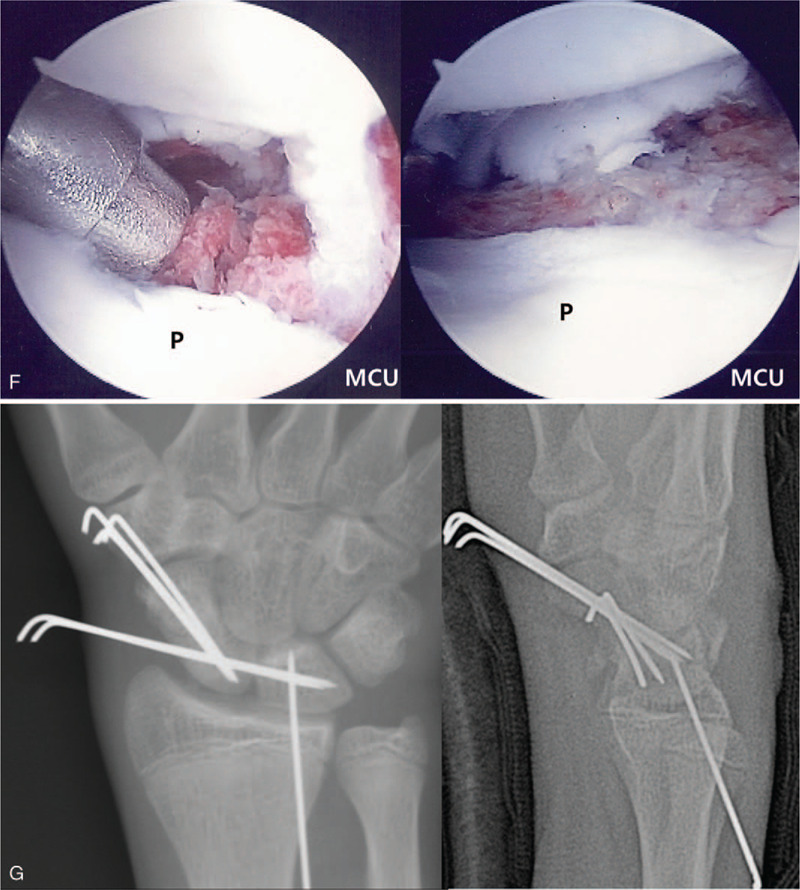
A 15-year-old boy patient with nonunion of the right scaphoid fracture. (A) Preoperative right wrist plain PA with ulnar deviation view showing nonunion at the waist of the scaphoid. (B) Preoperative right wrist sagittal CT scan shows nonunion at the waist of the scaphoid, about 88 degrees lateral intra-scaphoid angle and a humpback deformity. (C) Mid-carpal arthroscopy image of scaphoid nonunion site (P: proximal fragment, D: distal fragment). (D) Mid-carpal arthroscopy image of nonunion site after debridement showing punctate bleeding from proximal and distal fragments. (E) Mid-carpal arthroscopy image of SL joint showing volar side instability (Sc: scaphoid, Lu: lunate). (F) A mid-carpal arthroscopy image of percutaneous autogenous iliac cancellous bone grafting at the nonunion site using cannula and trocar (left) and complete the bone graft (right). (G) Immediate postoperative plain right wrist PA with ulnar deviation and lateral radiographs show internal fixation with K-wires and grafted bone at the nonunion site. (H) Postoperative 9 weeks K-wires removal axial and sagittal CT scans show complete bony union and correction of humpback deformity. (I) At 54 months’ follow-up plain right wrist PA with ulnar deviation and lateral radiographs show solid bony union.
